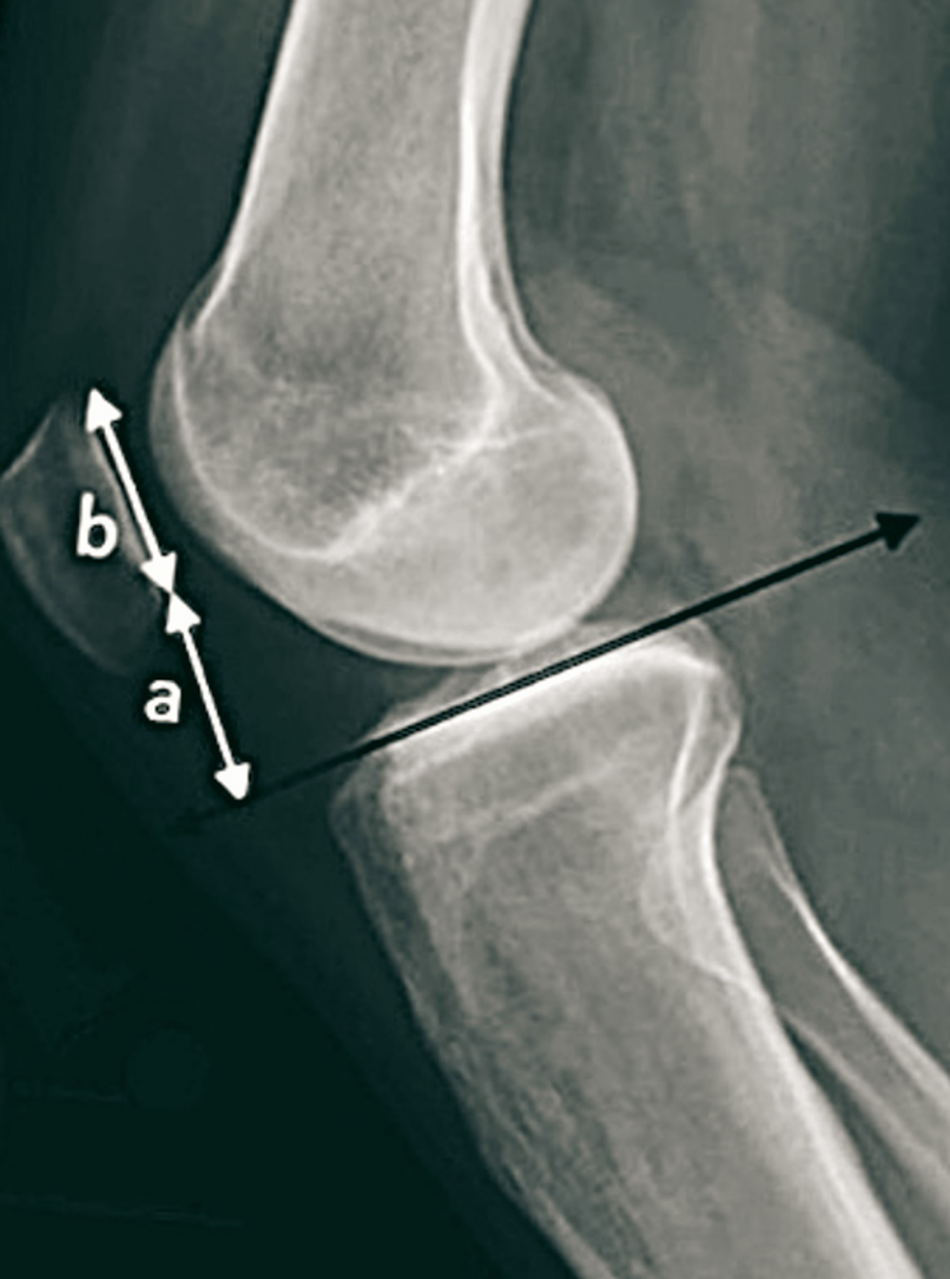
Radiographical demonstration of the Blackburn-Peel ratio (A/B) on a lateral knee radiograph.
